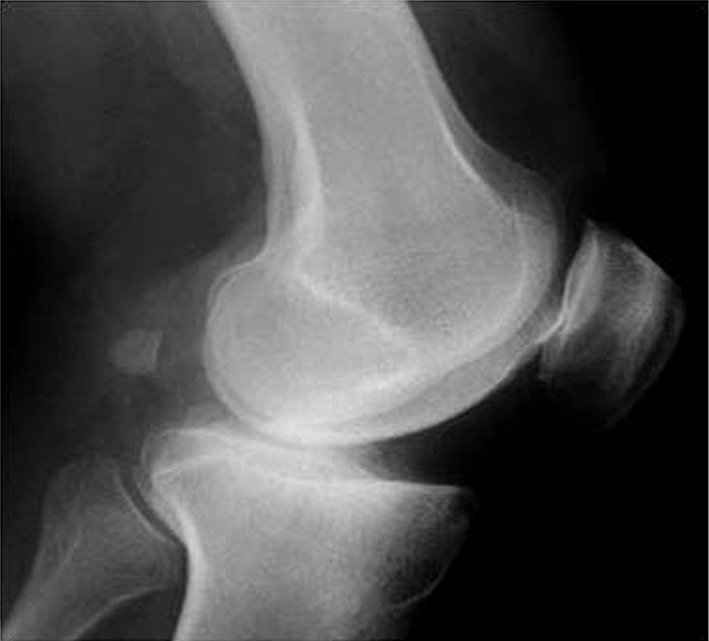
Lateral X-ray showing posterior wear of the tibial plateau in an anterior cruciate ligament (ACL)-deficient knee
